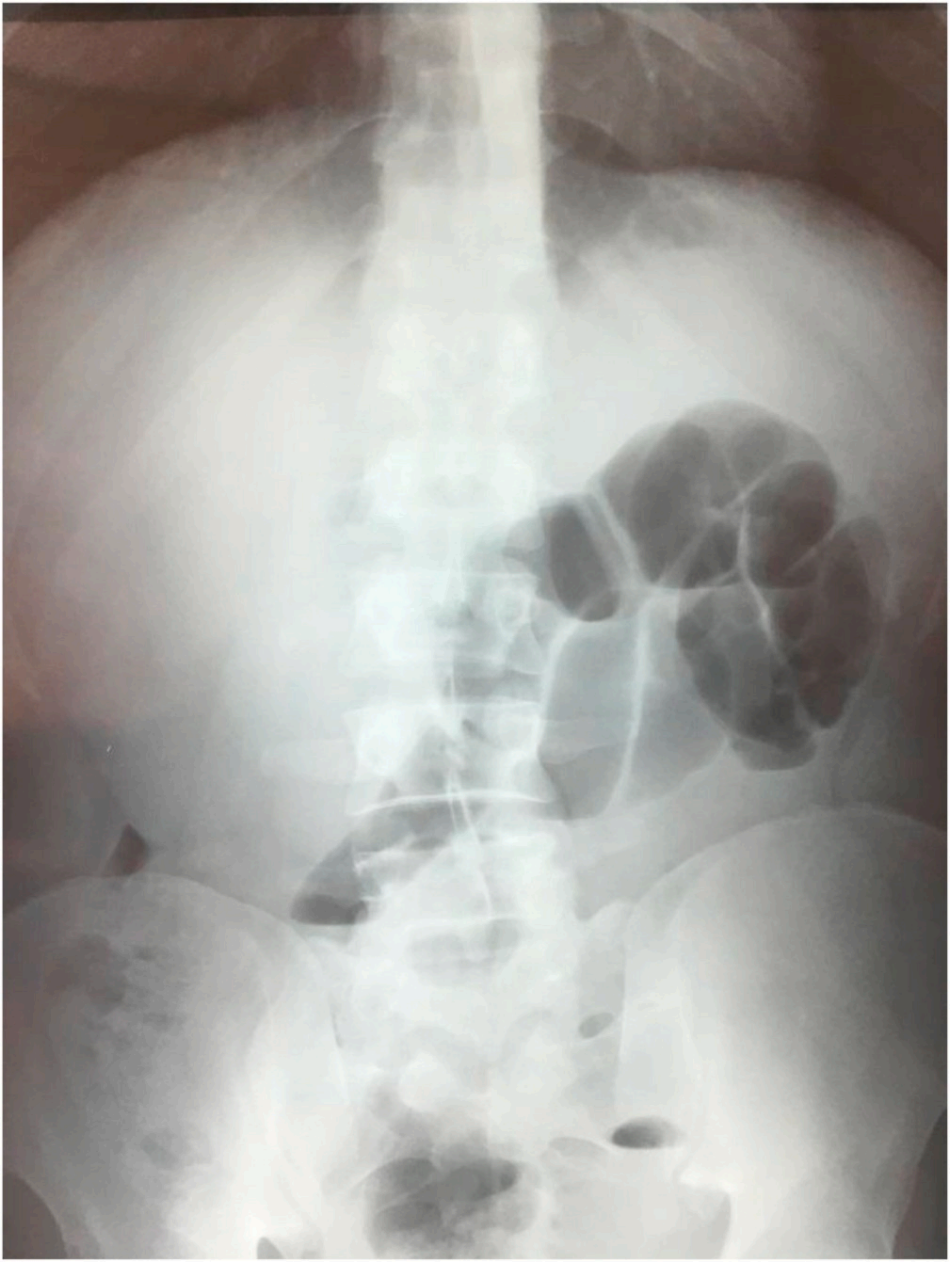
X-ray of the abdomen without preparation showing hail water levels.
